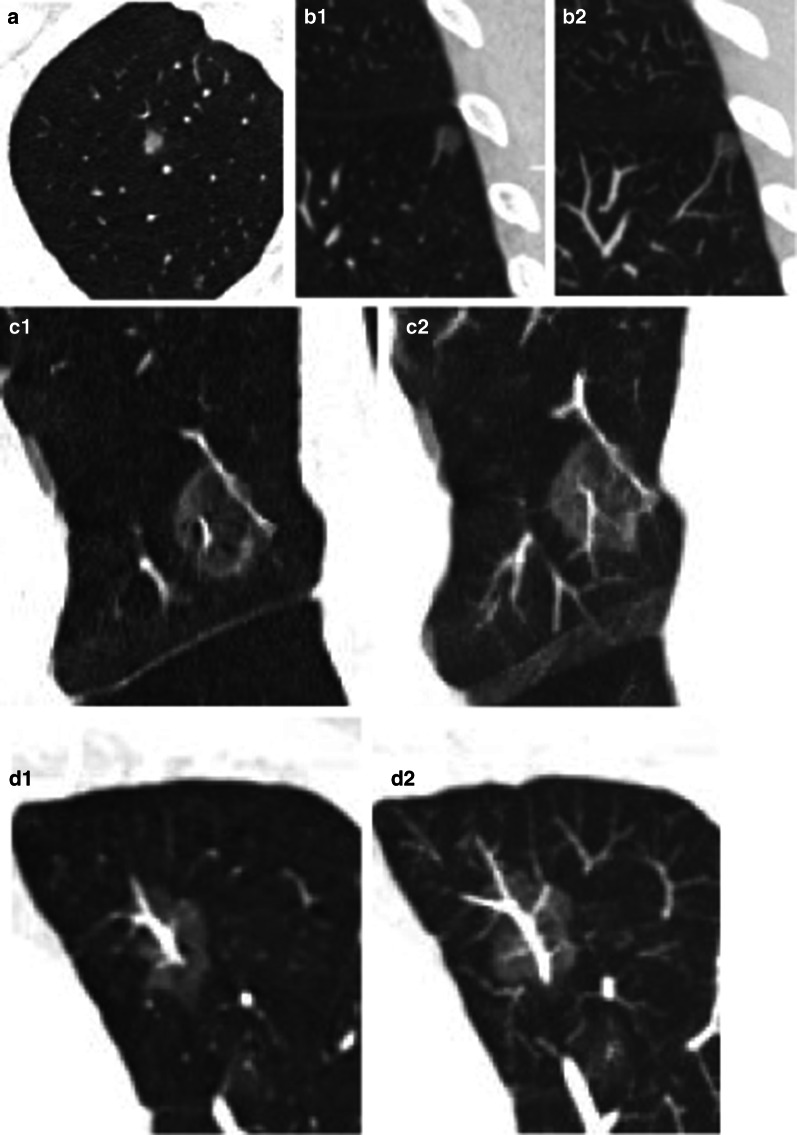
CT images of patients with malignant GGNs. A 6-mm GGN (atypical adenomatous hyperplasia) in the right upper lobe without internal vessels (a). A PA branch without change is detected in an 8.5-mm GGN [adenocarcinomas in situ (AIS)] in the left lower lobe (b1: b2). Two vessels (1 PA and 1 PV) were detected in a 17.5-mm GGN (AIS) in the left upper lobe (c1: c2), the PV was thickened with rigid course. An irregularly thickened PV branch is detected in a 19.5-mm GGN (IA) in the left upper lobe (d1: d2)
